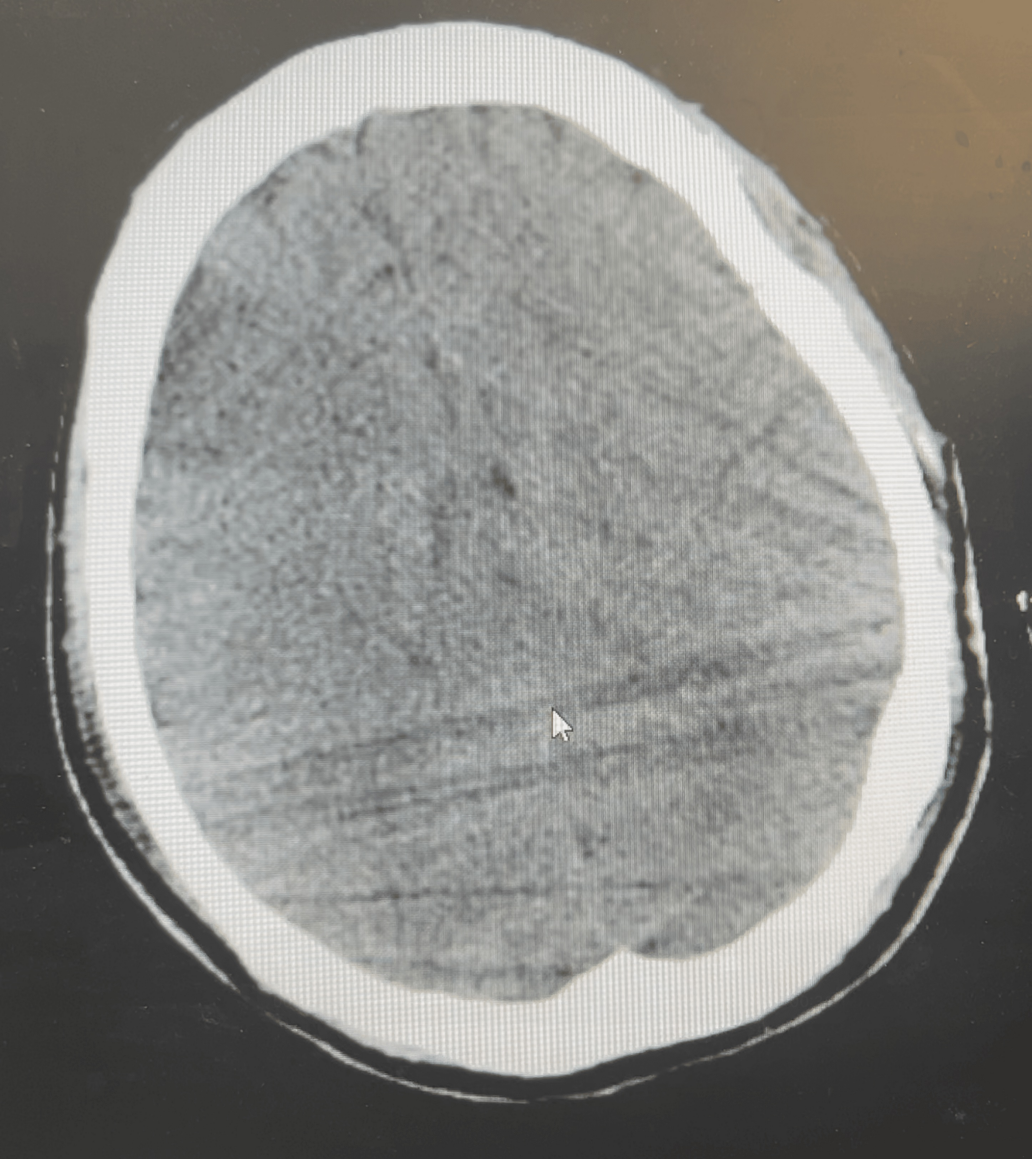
Axial non-contrast CT of the brain demonstrating diffuse cerebral hypoattenuation with loss of gray-white matter differentiation and sulcal effacement, consistent with global cerebral edema in the post-cardiac arrest setting.
